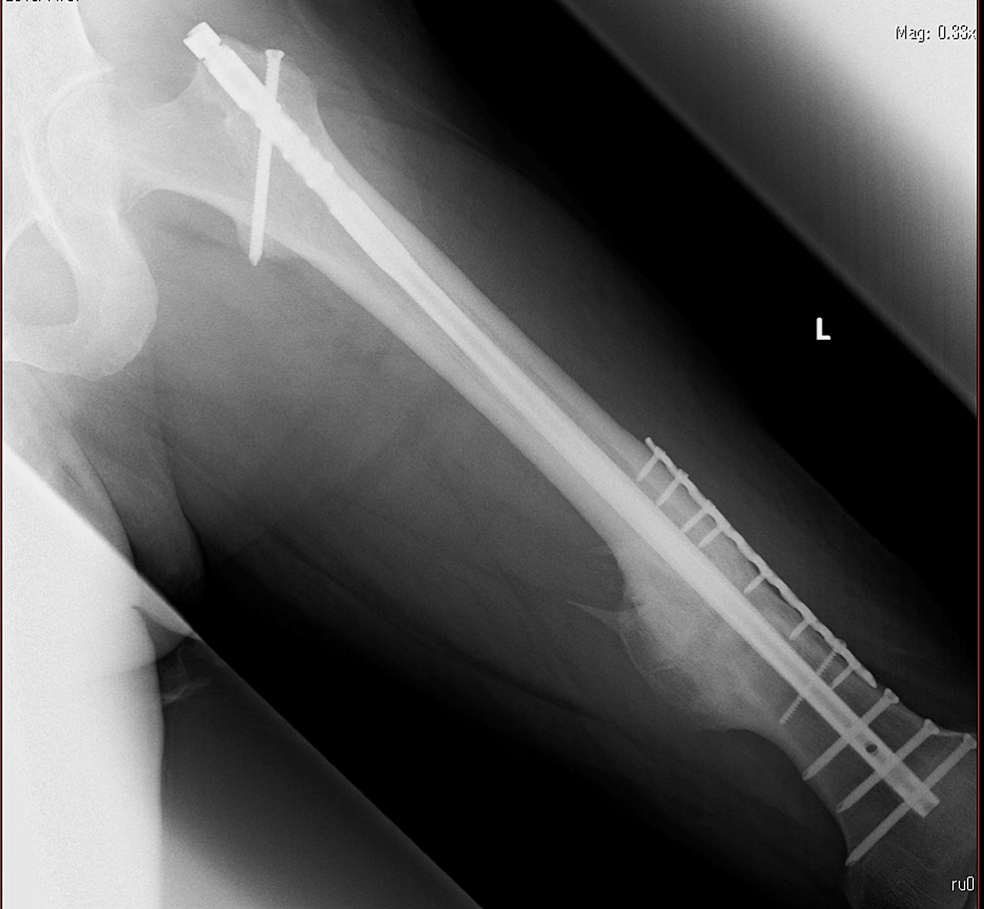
Left femur preoperative X-ray Bone fusion has been achieved.
